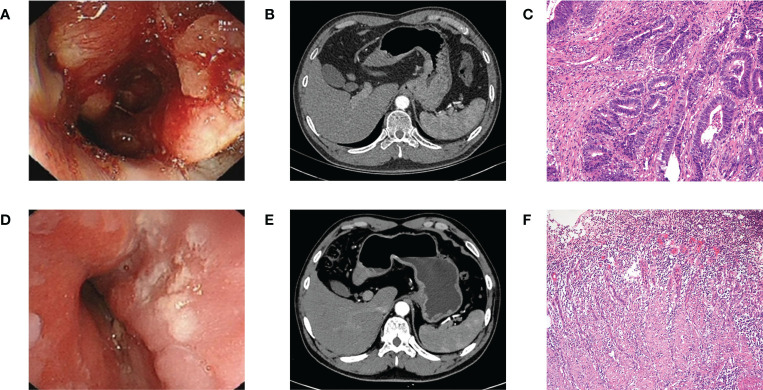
(A) Endoscopy before NACT plus tislelizumab. (B) CT before NACT plus tislelizumab. (C) Pathology before NACT plus tislelizumab. (D) Endoscopy after NACT plus tislelizumab. (E) CT after NACT plus tislelizumab. (F) Pathology after NACT plus tislelizumab. NACT, neoadjuvant chemotherapy.
